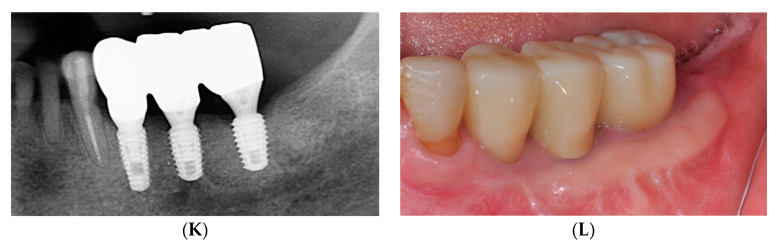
(A) Occlusal, (B) buccal, and (C) palatal view at the initial examination 3 months after implant’s first-stage surgery. (D) Creating recipient bed. (E) Healing abutment installation. (F) The harvested gingival graft. (G) A prefabricated acrylic wafer using a vacuum former. (H) The acrylic wafer placed at the donor site. (I) The gingival graft secured by two fixation tacks. (J) One week after simplified free gingival graft (sFGG). (K) Panoramic radiograph and (L) clinical condition one year after sFGG.
